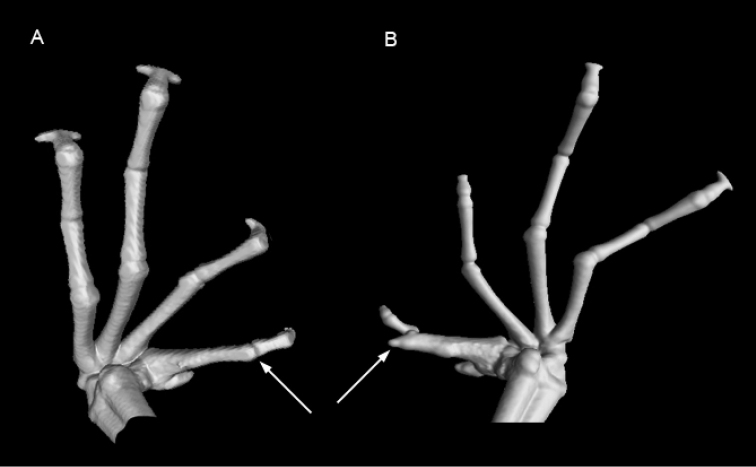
CT-scan image of the dorsal side of the hands of *Petropedetes
